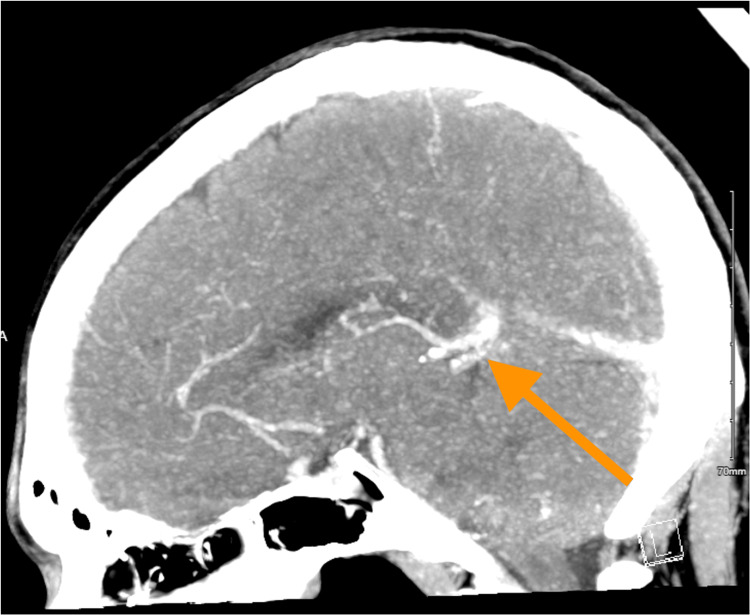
CT venogram demonstrating nonocclusive central venous sinus thrombosis (orange arrow) CT: computed tomography
